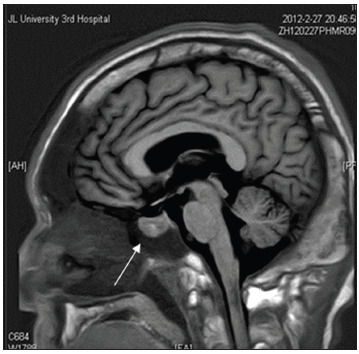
Findings from cerebral magnetic resonance imaging (MRI). An enlarged pituitary gland (arrowhead) appeared as a mass-like region with heterogeneous signal intensity and focal hyperintensity.
